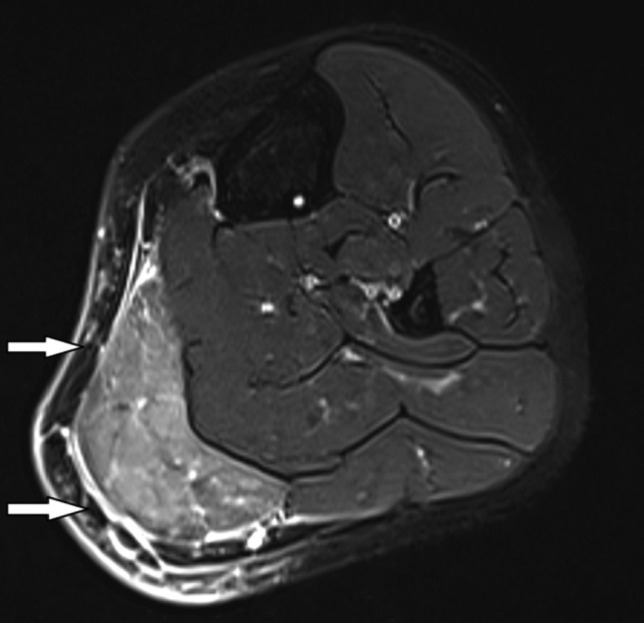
Left leg T2 weighted MRI coronal image showed increased signal intensity on T2 weighted image and diffuse enhancement in gastrocnemius muscle (white arrow).
